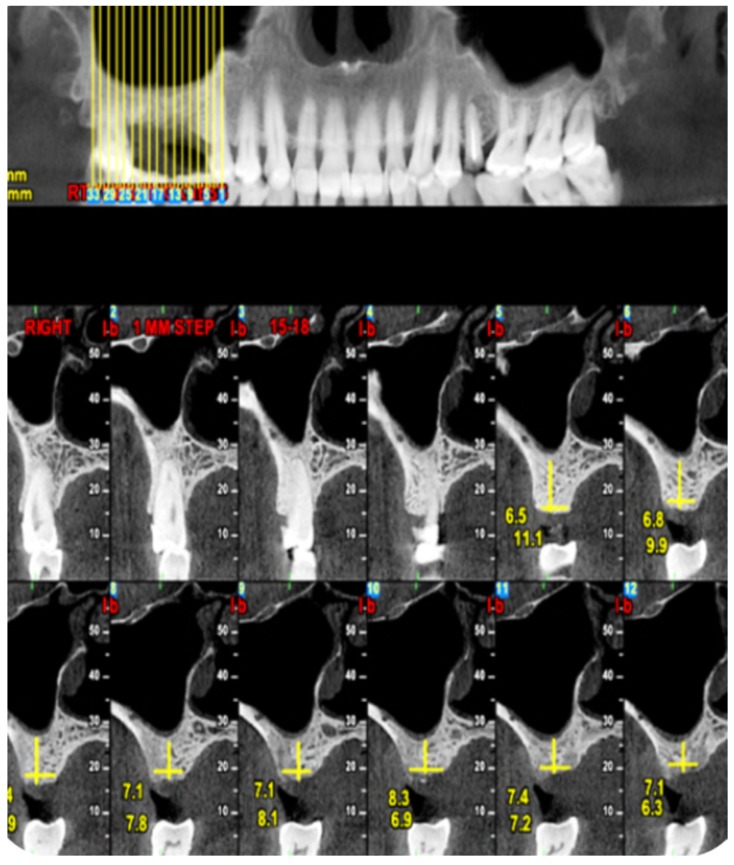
Preoperative CT view demonstrating residual alveolar ridge height.
